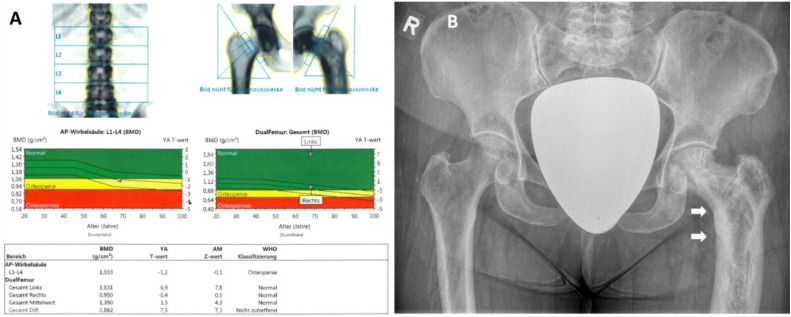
A, Dual-energy X-ray absorptiometry demonstrating extreme site discordance with markedly increased areal BMD at the left total hip compared with the right hip, contrasted by osteopenic lumbar spine values. B, Pelvic radiograph highlighting focal osseous remodelling of the left proximal femur (arrows). BMD, bone mineral density.
